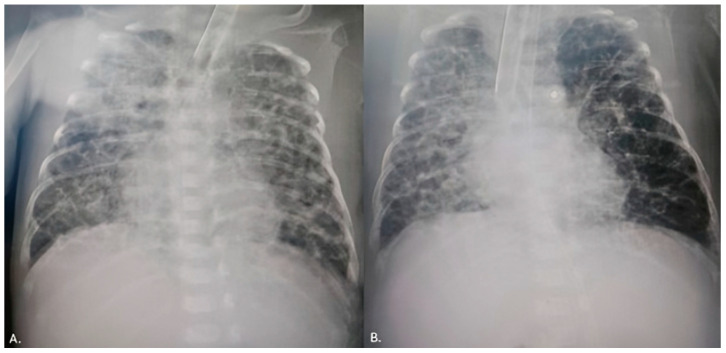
X-ray findings before (A) and after (B) transcatheter PDA closure.
